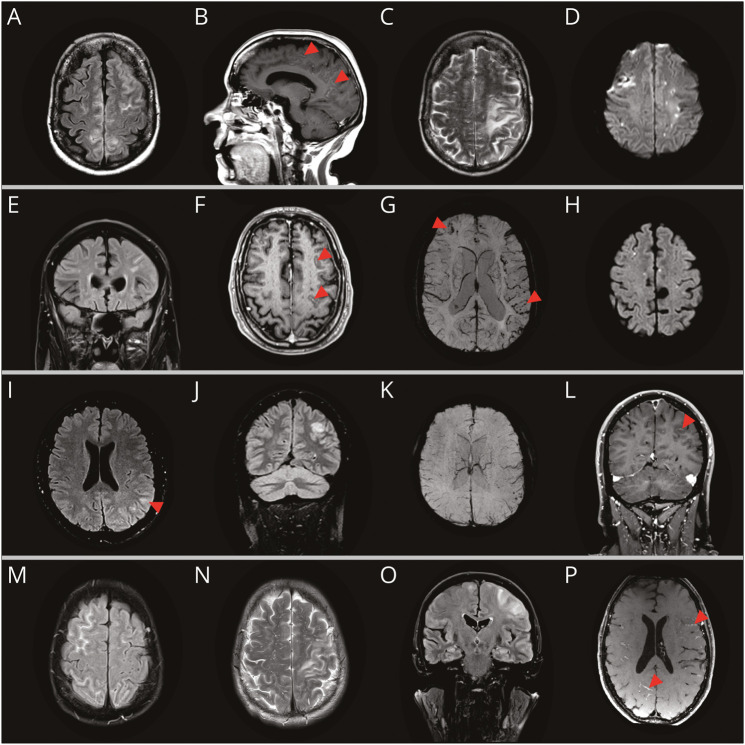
Representative MRI Findings for the Cases Described Case 1 (A–D): MRI at initial presentation showed sulcal hyperintensity (SH) on fluid attenuated inversion recovery (FLAIR) images (A), leptomeningeal contrast enhancement (B, red arrowheads); MRI at relapse 5 months after initial presentation showed asymmetric white matter hyperintensity (WMH), over the left parietal and occipital lobes (C), in addition to multiple cortical and subcortical foci of restricted diffusion on DWI sequences (D). Case 2 (E–H): MRI at presentation showed SH over the left frontal lobe on FLAIR images (E), leptomeningeal contrast enhancement (F, red arrowheads), lobar cerebral microbleeds (CMB, red arrowheads), and areas of cortical superficial siderosis (cSS), mostly over the left frontal lobe on SWI (G), and multiple subcortical DWI lesions (H). Case 3 (I–L): MRI at initial presentation showed a subtle area of cortical swelling and SH in the left parietal region (I, red arrowhead); MRI at relapse showed a new patch of the WMH in the left temporoparietal region (J), associated with a cluster of CMB (K). The follow-up image 2 years later showed leptomeningeal enhancement in the left temporoparietal region (L, red arrowhead). Case 4 (M–P): MRI at initial presentation showed SH with gyral swelling on the FLAIR image over the right frontal lobe and occipital lobes bilaterally (M); MRI at relapse 9 months later showed areas of WMH with mild parenchymal swelling on T2 (N) and FLAIR (O) images primarily affecting the left perirolandic region, and leptomeningeal contrast enhancement (P, red arrowheads).
